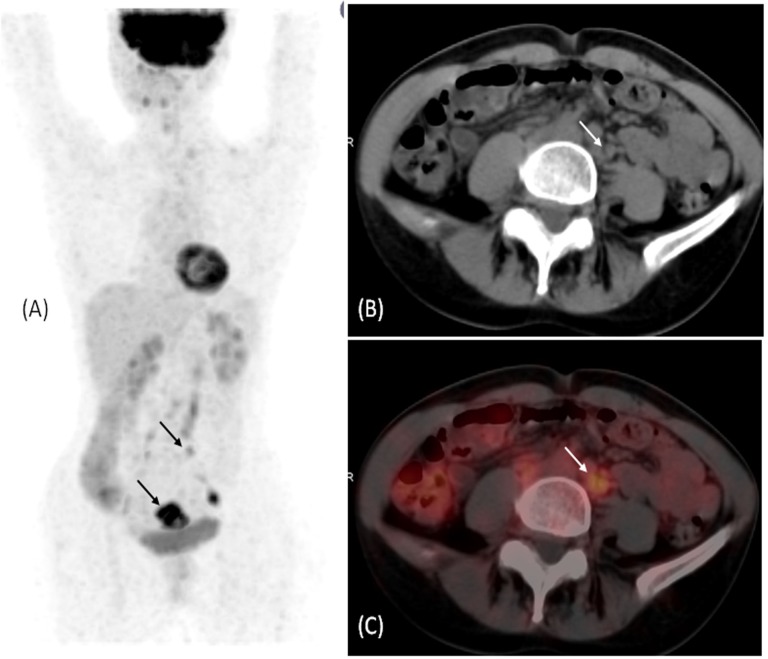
A 60 year-old female patient with cervical cancer underwent ^18^F-FDG PET/CT for staging. (A) Maximum intensity projection (MIP) of the ^18^F-FDG PET demonstrated the hypermetabolic cervical tumor in the pelvis (arrow) with multiple foci of abnormal increased FDG uptake in the pelvis and abdomen (arrow). (B) Transaxial CT scan demonstrated subcentimetric retroperitoneal lymph nodes (arrow) not categorized as pathological based on morphologic criteria. (C) Transaxial fused ^18^F-FDG PET/CT image in the abdomen demonstrated multiple hypermetabolic retroperitoneal lymph nodes (arrow) correlating to the subcentimetric lymph nodes seen on CT scan and the focal abnormal increased FDG uptake seen on MIP
